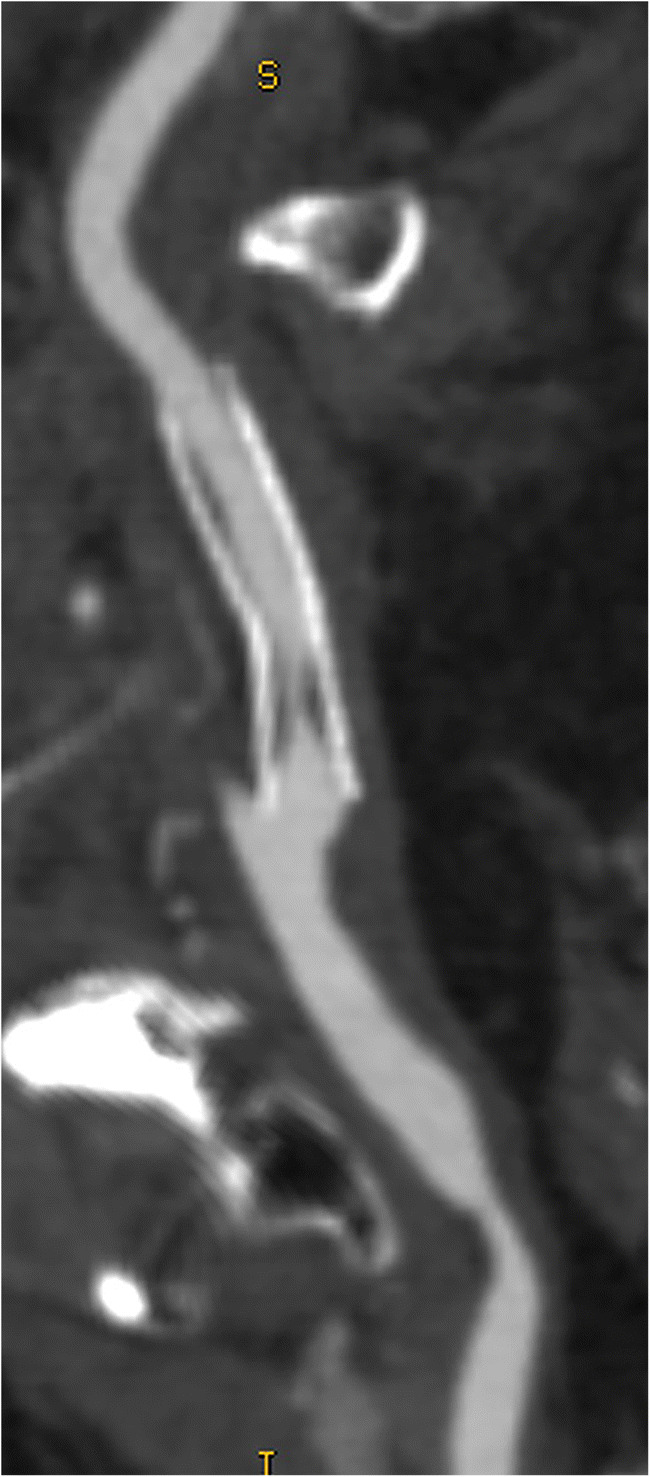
Curved MPR image of neo-intimal hyperplasia in a carotid stent, producing significant proximal luminal narrowing. CT offers exquisite anatomical detail, highlighting complications like the mentioned intima hyperplasia and guiding as such further treatment and potential re-intervention
